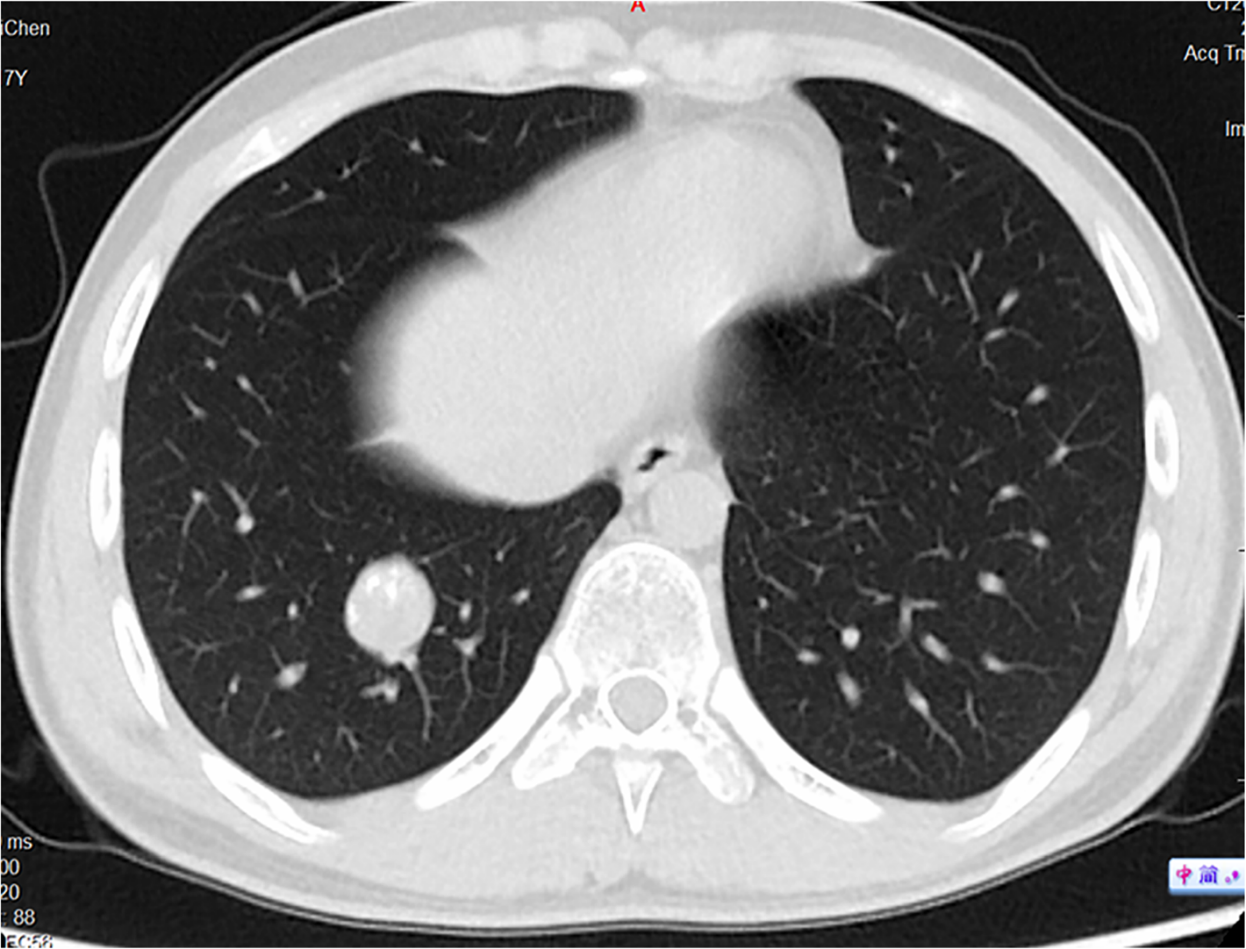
A missing nodule progressed on chest CT (red circle) after radiation. (Eleven months later, this nodule, which had been missed for local therapy, became larger and obvious, and 3 new lesions also appeared on this patient’s chest CT, all of which were later subjected to a second cycle of radiotherapy. At the last follow-up, the patient was still disease free. CT = computed tomography)
